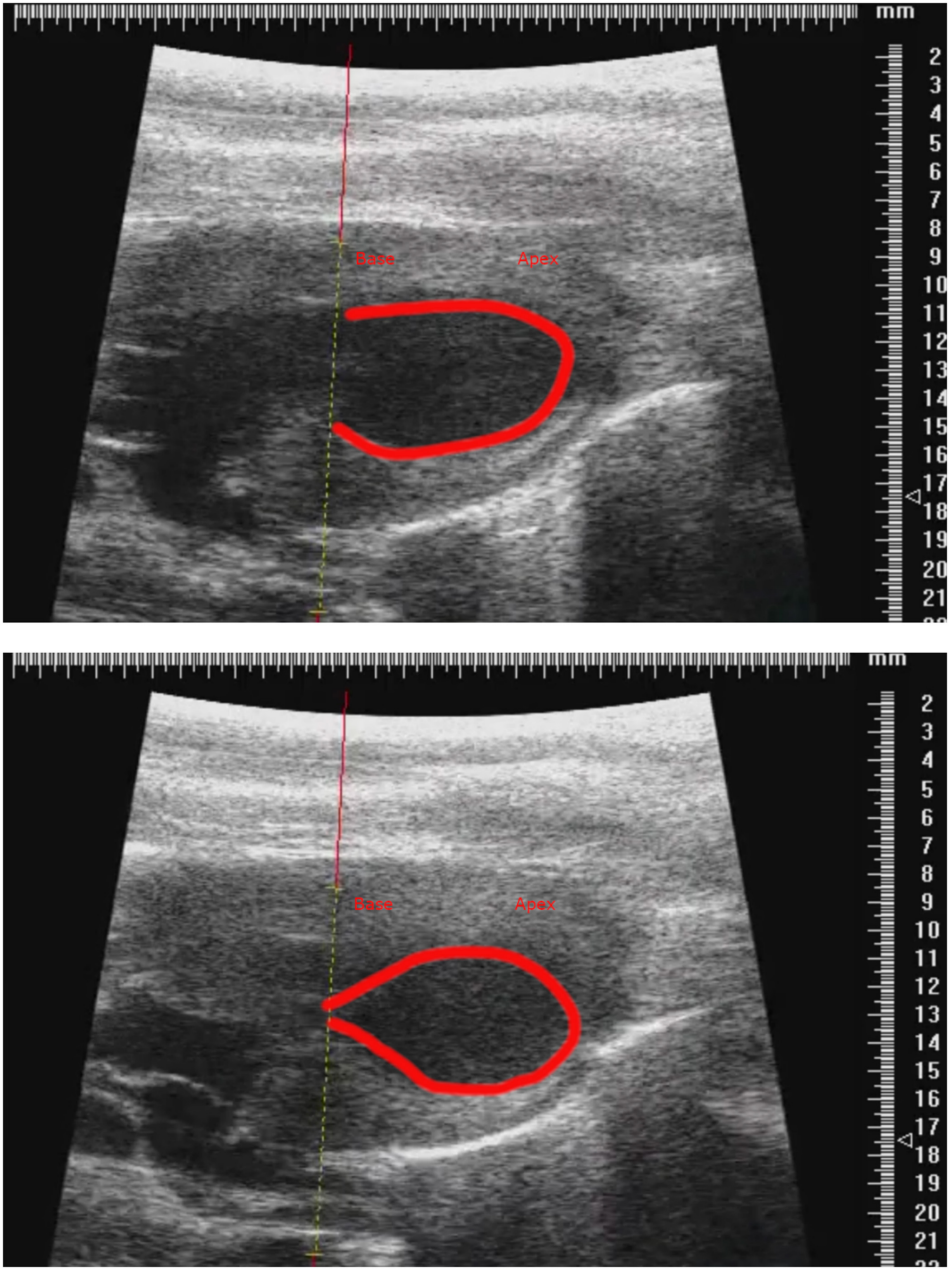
Representative parasternal long axis B-mode images of end-diastole (top) and end-systole (bottom) after induction of TTS-like contractility (base: left; apex: right), with trace (red line) drawn around endocardial border. Scale in mm.
